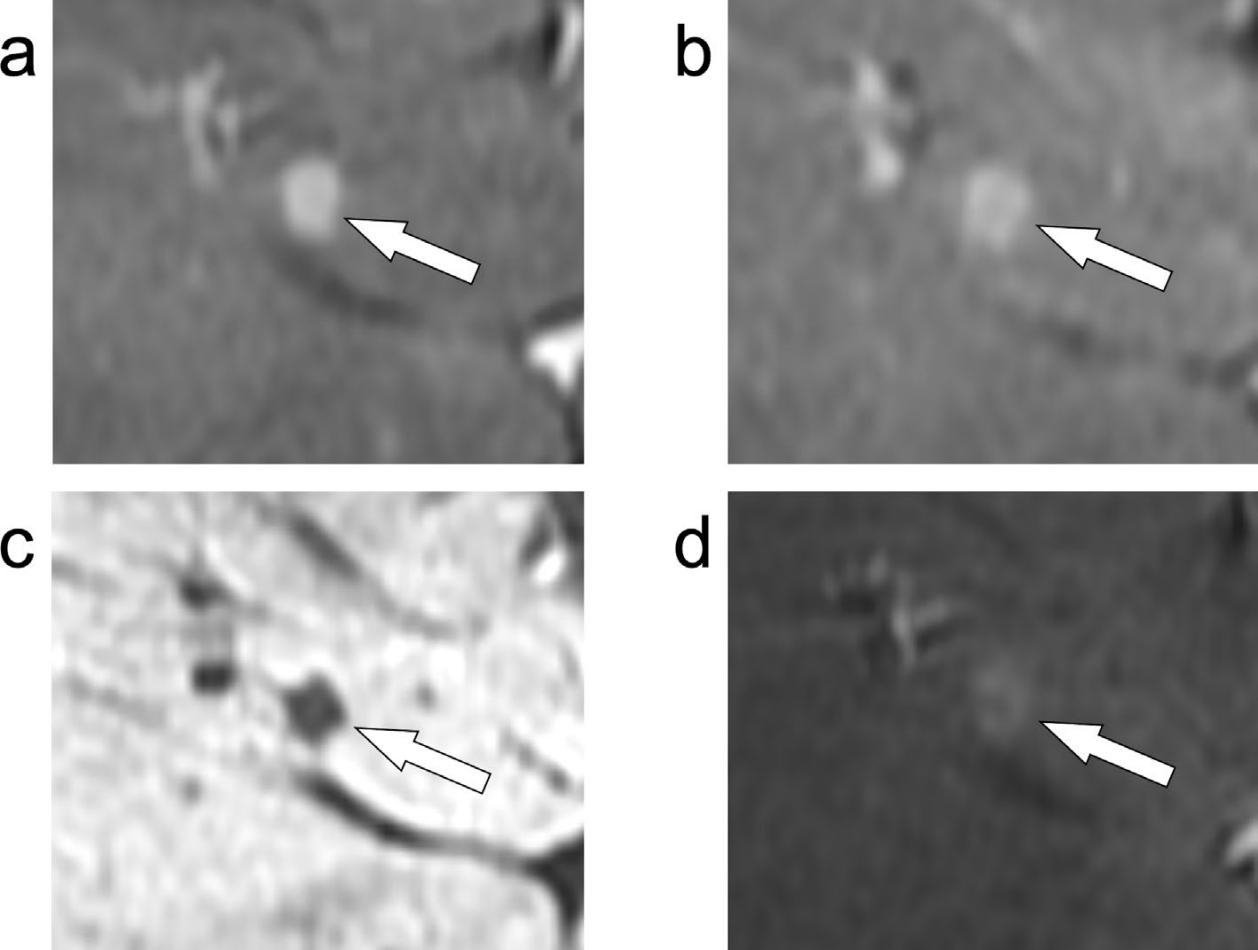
Axial T_1_‐weighted fat‐suppressed imaging of the liver demonstrates an observation with arterial phase hyperenhancement (a, arrow) without nonperipheral washout or an enhancing capsule on the portal venous phase (b, arrow) that fulfills LR‐3 criteria (intermediate probability of malignancy) based on the major features. The observation demonstrates hepatobiliary phase hypointensity (c, arrow) which is an ancillary feature favoring malignancy in general, not HCC in particular, which could be used to increase the category to LR‐4 (probably HCC). However, a prior MRI from over 3 years earlier demonstrated this observation to be stable in size (d, arrow). Size stability > 2 years is an ancillary feature favoring benignity that negates the ancillary feature favoring malignancy. The final categorization of this observation remained LR‐3.
